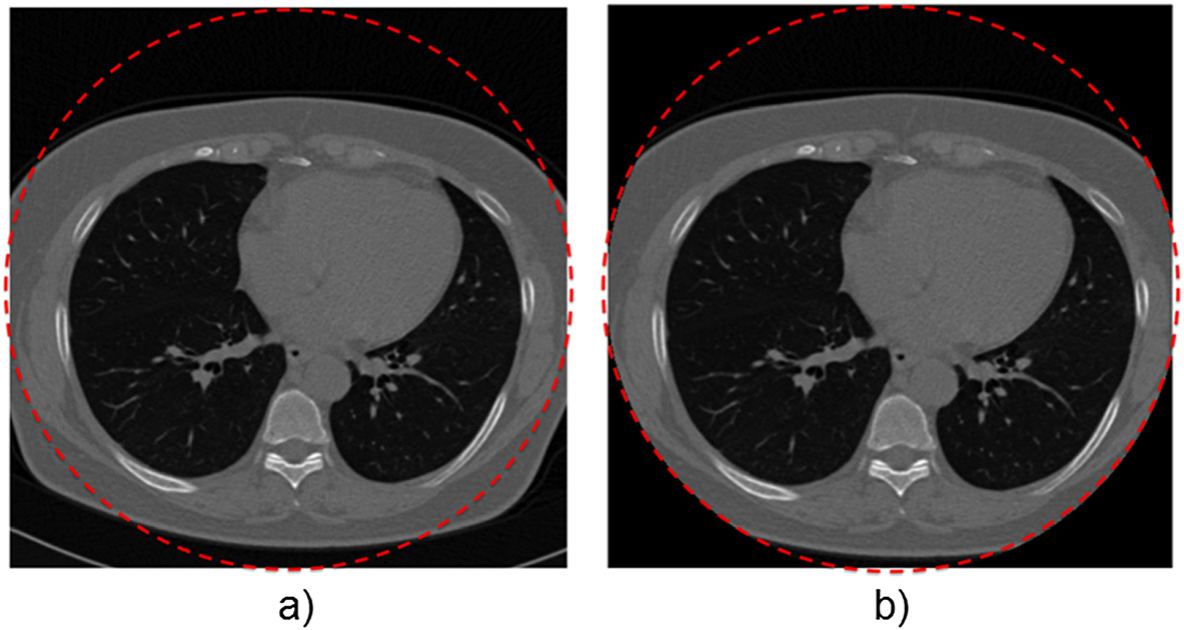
Extraction of the ROI in a CT image: a) Original CT image and b) CT image after extraction of the ROI. The images were obtained from two different public databases and different scanners were used for acquisition. Different forms and views can be observed. The extraction of the ROI is performed in order to eliminate the differences between images and to standardize all the CT scans. The final form is highlighted with a dashed red circle.
